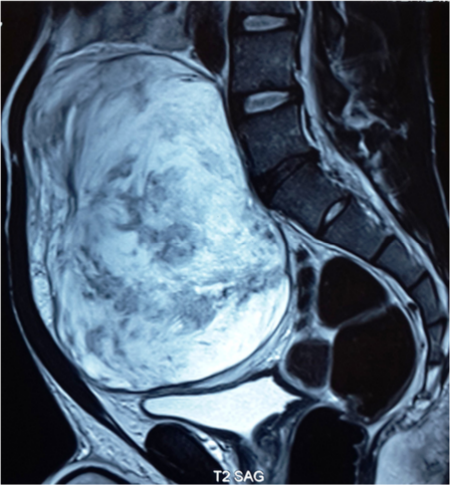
Pelvic magnetic resonance imaging showing a large, well encapsulated preperitoneal tumor.
